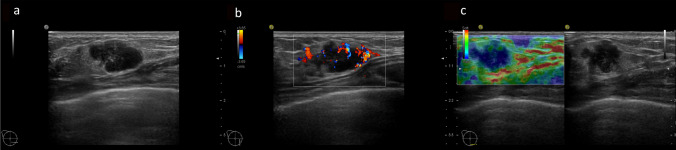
A 38-year-old woman with a *BRCA1* mutation and high-grade invasive ductal carcinoma (triple-negative subtype). Breast ultrasound demonstrates a circumscribed polygonal mass without calcification. This tumor has high vascularity and score 4 elasticity (no strain in the entire lesion)
